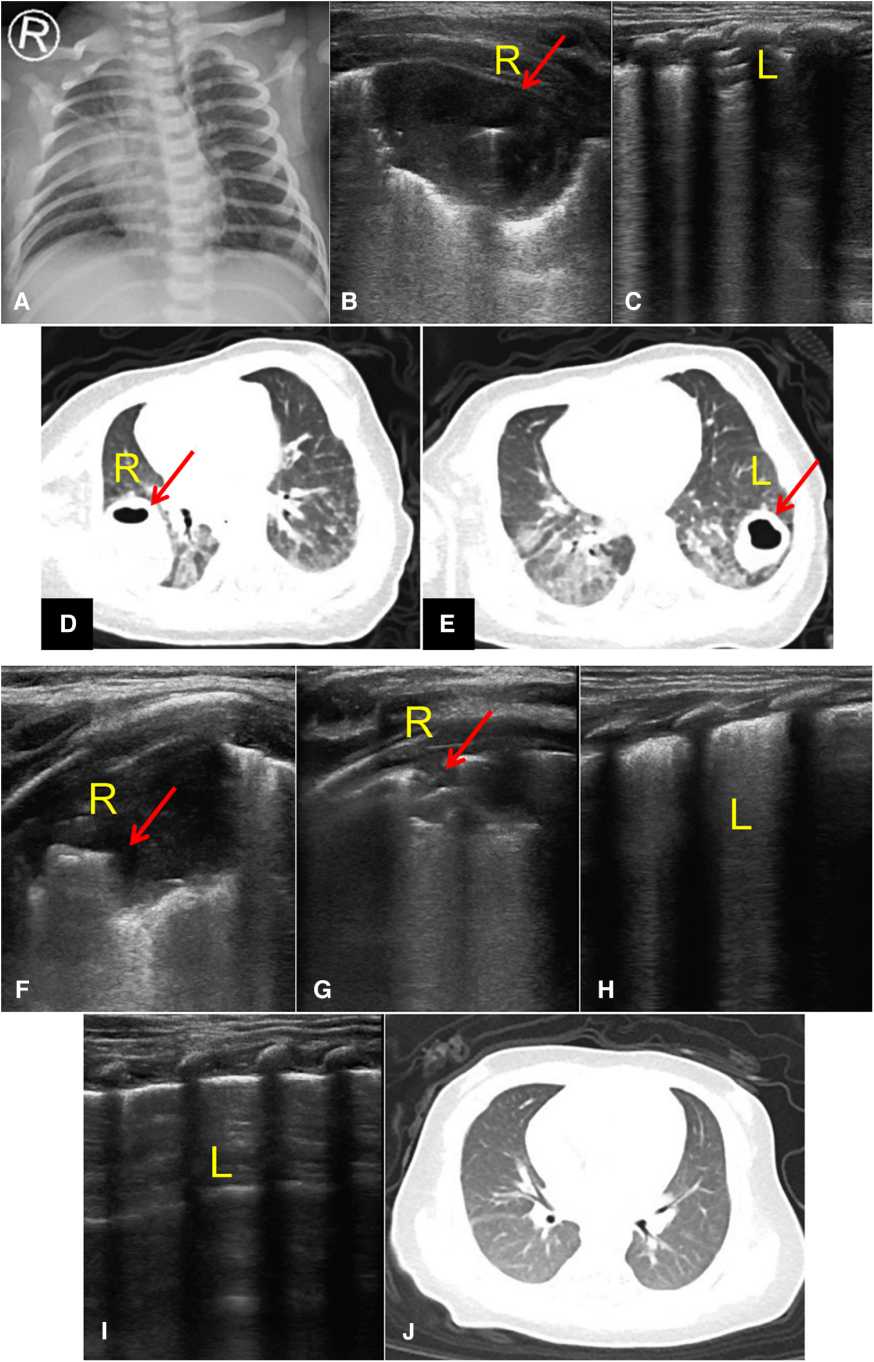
Post-treatment follow-up imaging results. (A) Chest x-ray showed an improvement in pneumonia compared to before. (B,C) Lung ultrasound: an irregular small consolidation (Red arrow, 25.8 mm^3^ × 12.2 mm^3^ × 10.3 mm^3^) in the posterior axillary area of the right upper lung, a small amount of bronchial inflation sign is visible, and no significant consolidation is detected in the rest of the right lung and left lung. (D,E) Chest CT scan: lumpy shadows in both lungs, with a right lung lesion size of approximately 15 mm^3^ × 20 mm^3^ × 28 mm^3^, with a left lung lesion size of approximately 20 mm^3^ × 17 mm^3^ × 10 mm^3^, smaller than before. (F–I) Lung ultrasound: an irregular small consolidation (Red arrow, 12.2 mm × 8.6 mm) in the posterior axillary area of the right upper lung, with a small amount of bronchial inflation sign visible. No significant consolidation was detected in the remaining areas of the right lung and left lung (J) Chest CT scan: patchy slightly high-density shadows in the upper and lower lobes of the right and left lungs, the lesions in both lungs are significantly absorbed and reduced compared to before, and the cavities disappear.
